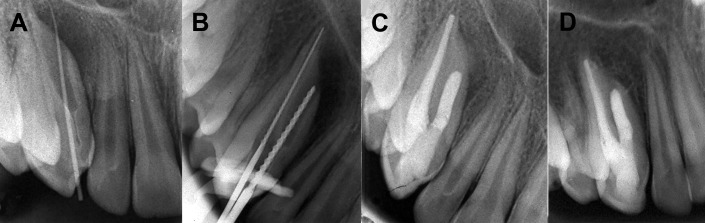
A: Periapical radiograph showing a gutta-percha point tracing the sinus tract and enamel like tract which is extended toward the invaginated portion. B: Length determination of the main and invaginated canals. C: Immediate postoperative periapical radiograph taken after final canal obturation and placement of coronal seal. D: 18 months follow-up radiograph demonstrating complete periapical healing.
